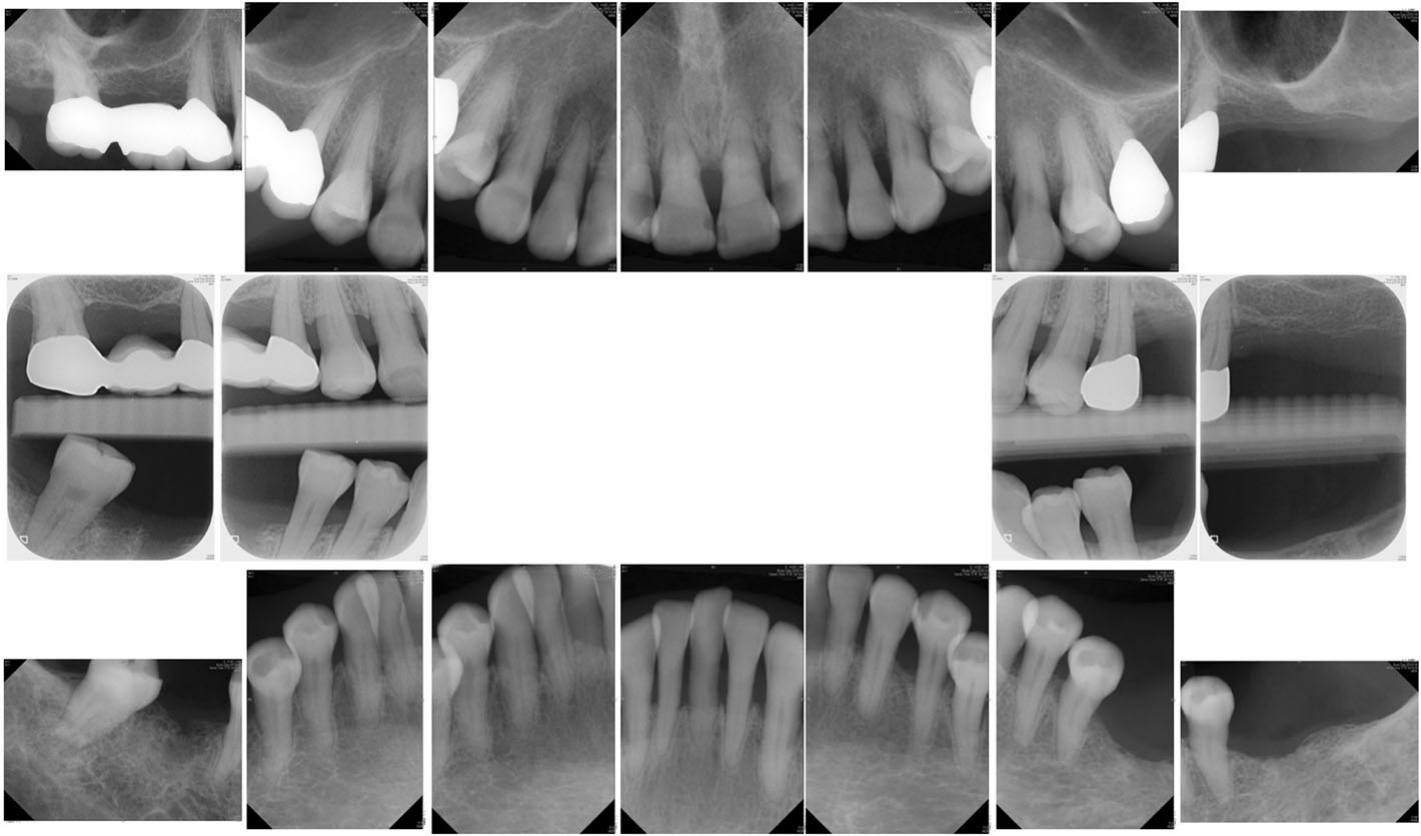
Two-year follow-up radiographs. The bone level was maintained throughout the treatment course
